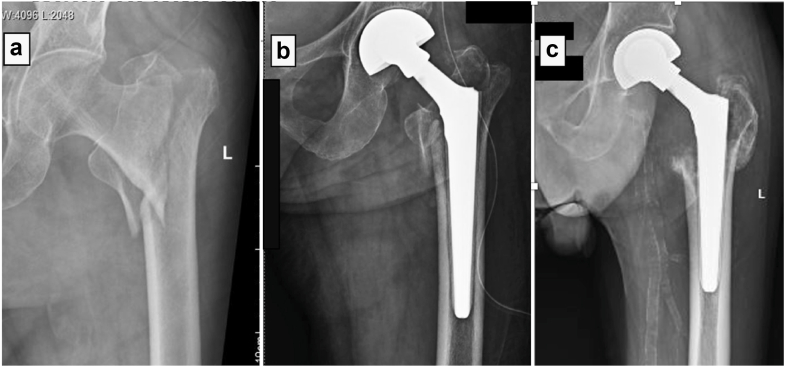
Radiographs of a patient who underwent bipolar hemiarthroplasty using a titanium, fluted, tapered cone prosthesis. (a) Preoperative radiograph of the involved side, AP view. (b) Immediate postoperative radiograph. (c) Radiograph at the latest follow-up. AP, anteroposterior.
